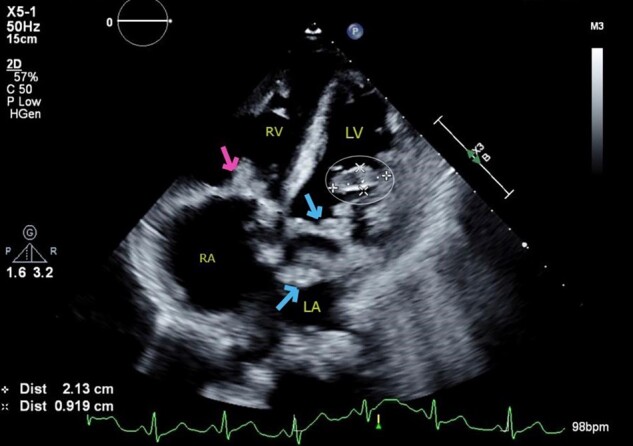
Transthoracic echocardiogram. Apical four chamber view showing cystic mass protruding from the anterolateral wall of the left ventricle into the cavity (cross and circle), along with multiple vegetations (vs. mass) on the mitral valve (arrows) and tricuspid valve (arrow).
